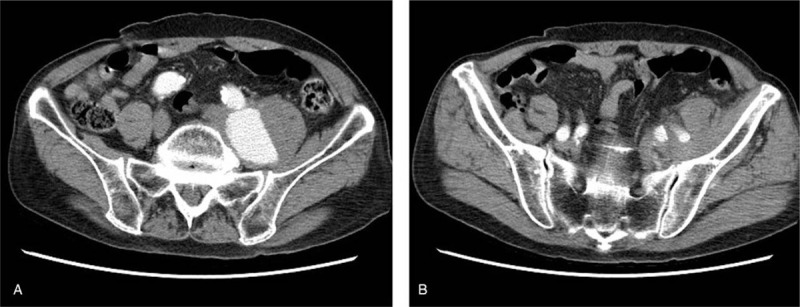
Computed tomography angiography (CTA) exam for pre-operation aorta evaluation. (A) Common iliac aneurysm with severe arterial calcification; (B) normal size of left internal iliac artery and external iliac artery.
